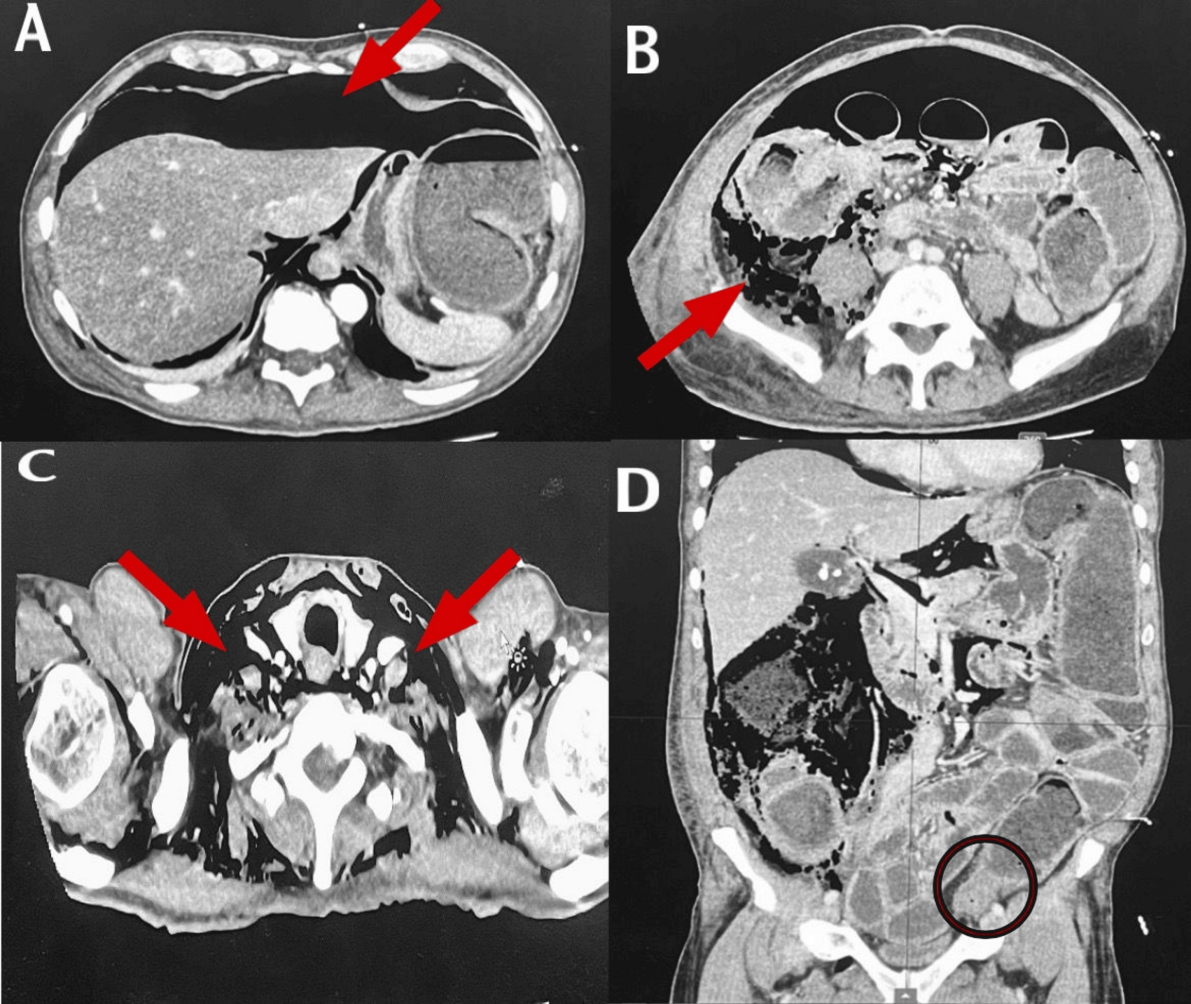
Computed tomography of the thorax, abdomen, and pelvis: (A) extensive pneumoperitoneum, (B) right retroperitoneal extension, (C) pneumomediastinum and extension in the neck, and (D) sigmoid stricture causing bowel obstruction
